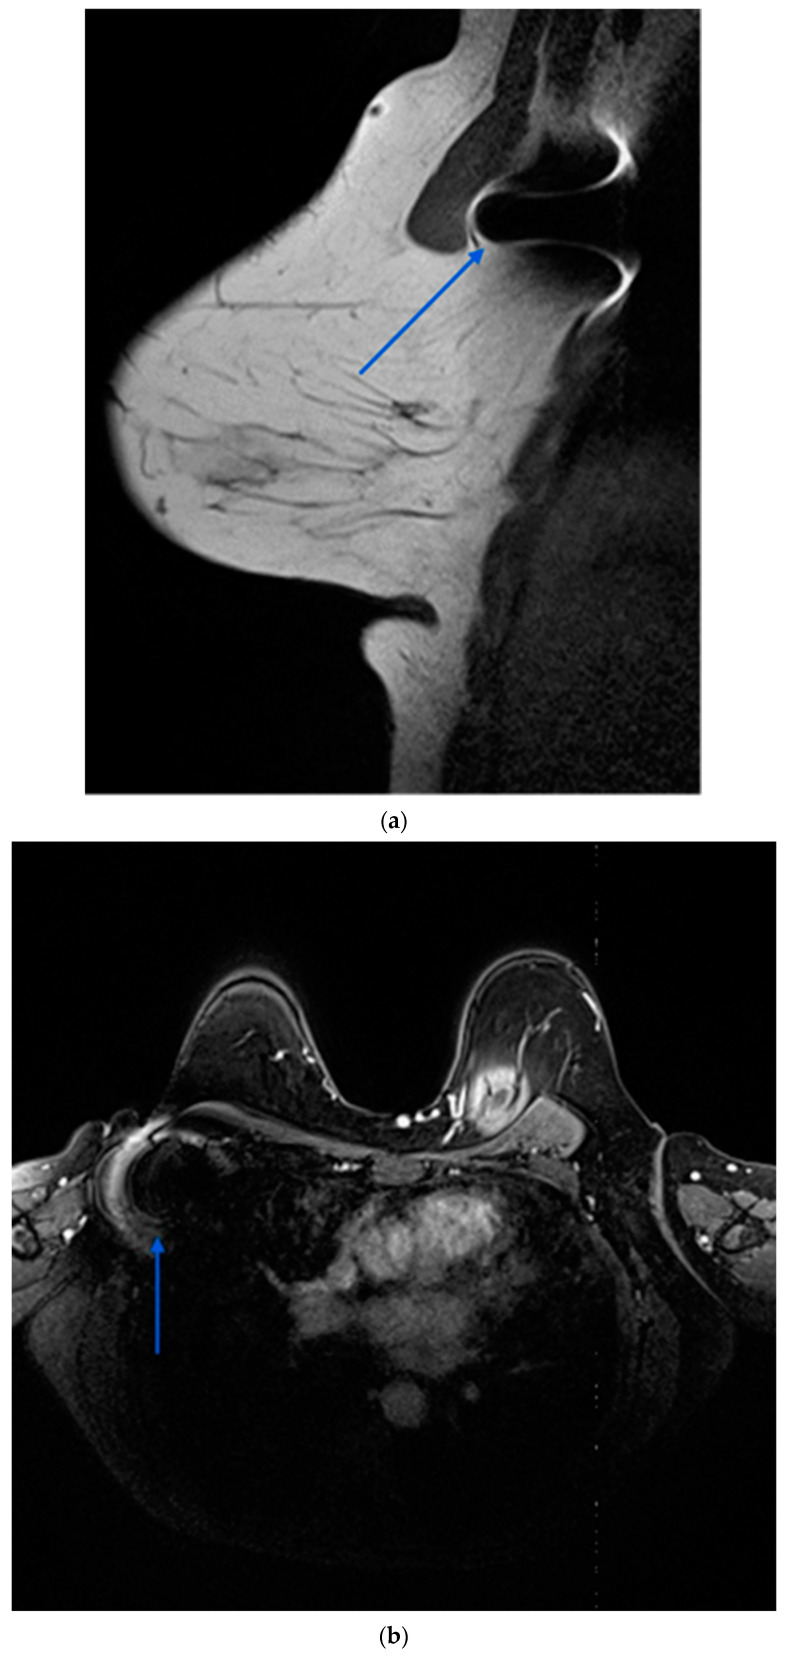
Contrast enhanced MRI after completion of NST demonstrating ferromagnetic artefact (blue arrows) generated by a magnetic seed previously placed in a right pathological axillary lymph node. (a): sagittal view; (b): axial view.
